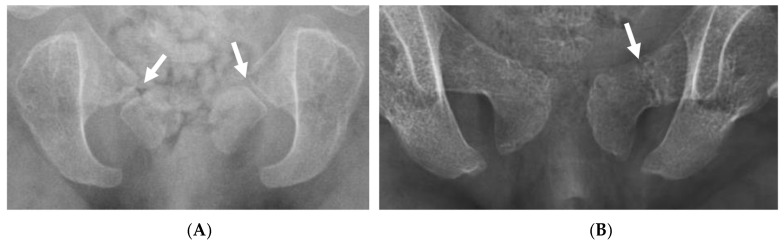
Enlarged anteroposterior pelvic radiographs of 3-year-old (A) and 4.5-year-old (B) patients with SEDC demonstrating bilateral (A) and unilateral (B) multiple ossification centers as vertical radiolucent lines dividing the ramus (white arrows).
